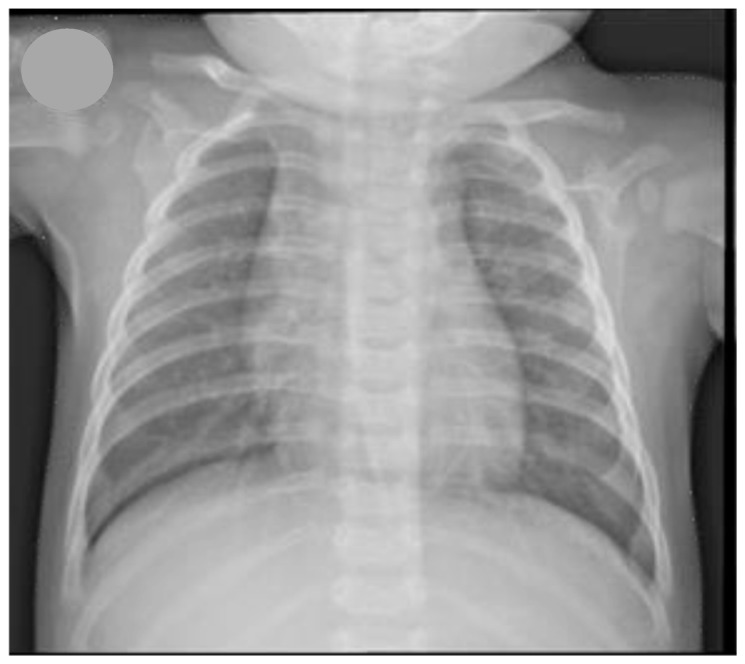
Chest X-ray showing a diffuse over-inflation of the lungs.
